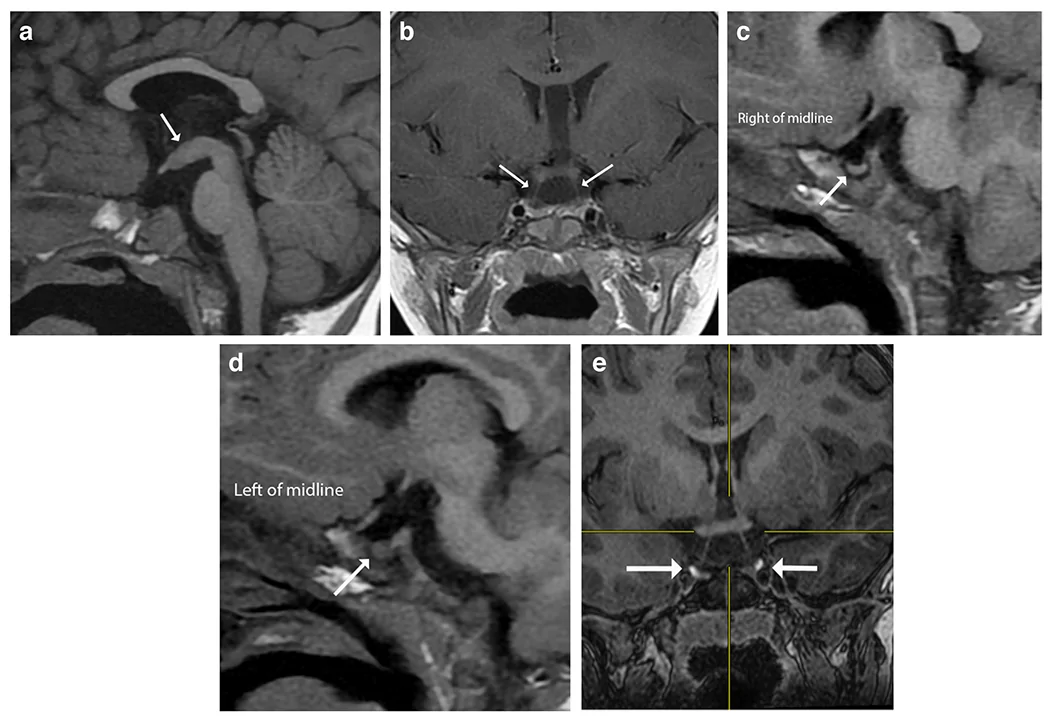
One-year-old female with short stature, originally presenting with a resected oral teratoma and head imaging unexpectedly identifying tubulomammillary fusion in the setting of a duplicated pituitary. a Sagittal T1-weighted sequence through the midline shows a uniform tubular thickening of tissue extending anteriorly from the midbrain along the hypothalamus in a manner that may mimic a hamartoma (*arrow*). b Coronal T1-weighted sequence through the sella shows duplicated, bilateral pituitary infundibula (*white arrows*, b). c, d Sagittal T1-weighted images through each hemi-sella show two distinct adenohypophyses (*arrows*). e Reconstructed coronal T1-weighted image through the hypothalamus, pituitary stalks, and bilateral pituitary bright spots (*arrows*, e). Laboratory studies followed over many subsequent years have been within normal ranges
